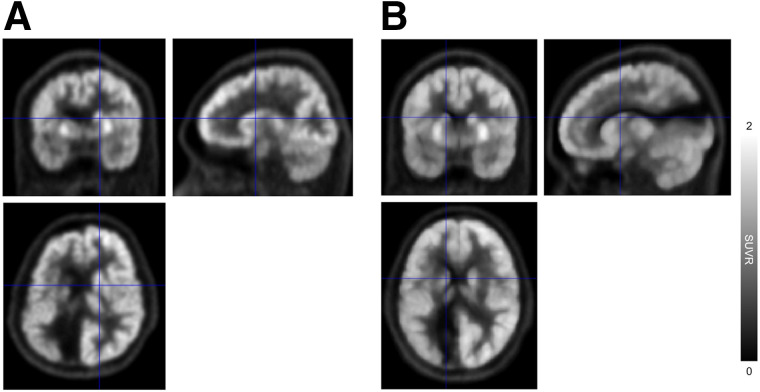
^18^F-FDG PET images of 21-y-old man with hypointense areas that are well preserved in terms of relative size and shape after spatial normalization. Images are shown in individual space before spatial normalization (A) and standard space after spatial normalization (B) using proposed method.
